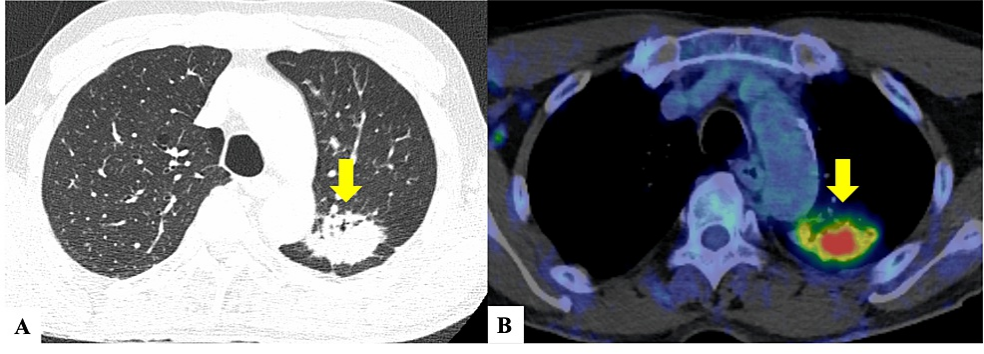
CT scans (A) Chest plain CT shows an irregular mass shadow measuring 47 mm in the upper lobe of the left lung (arrow). (B) On FDG-PET/CT, high FDG uptake with an SUVmax of 9.8 in the tumor of the left upper lobe (arrow). FDG: fludeoxyglucose-18; PET: positron emission tomography
